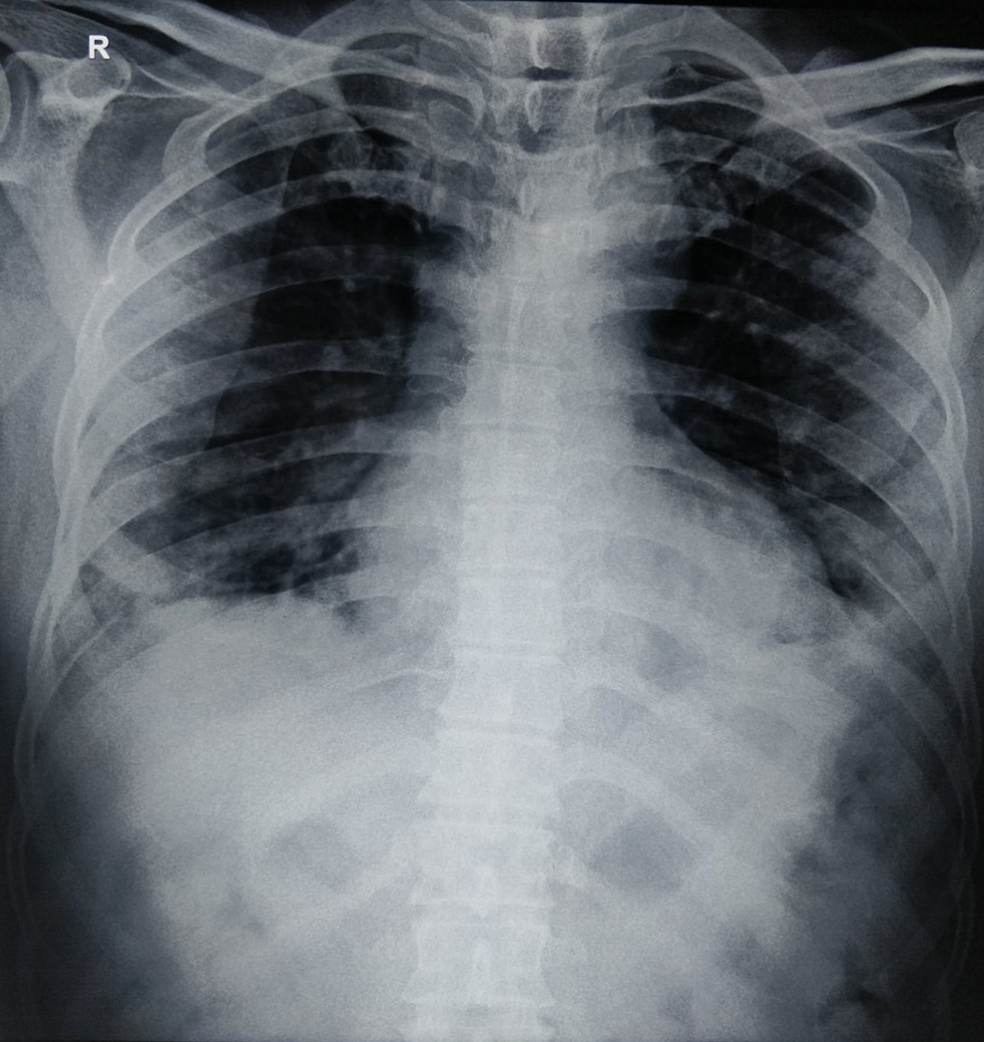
Chest X-ray after two days of administration of tocilizumab.
